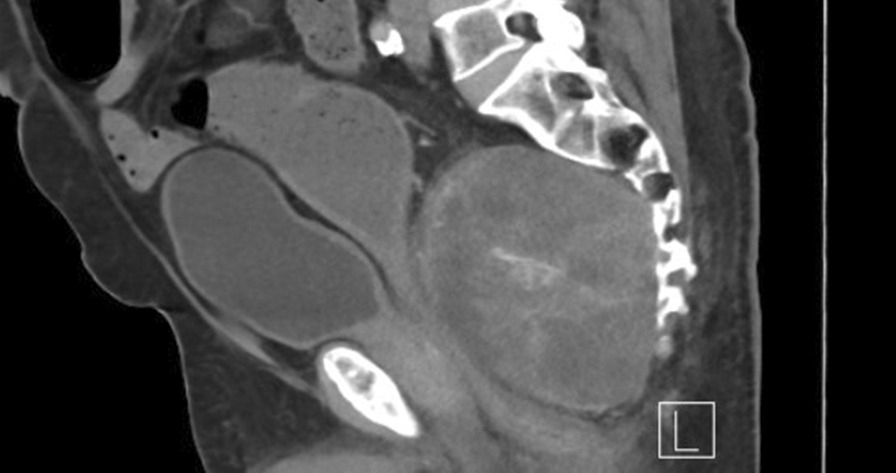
Lumbar computed tomography indicated a large midline, destructive, osseous, sacrococcygeal mass with a large presacral mass with soft tissue components and linear calcification measuring 12.5 × 10.4 × 10.6 cm
